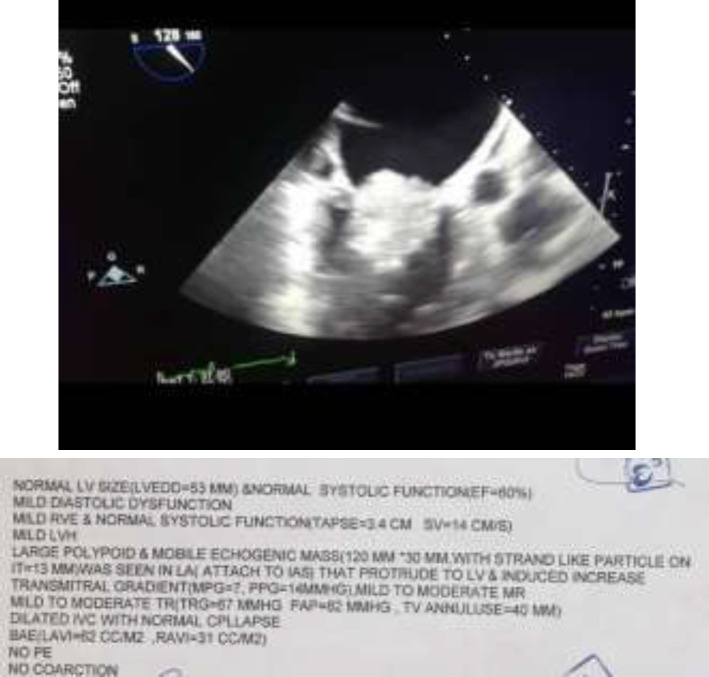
Echocardiography of the patient with Myxosarcoma
